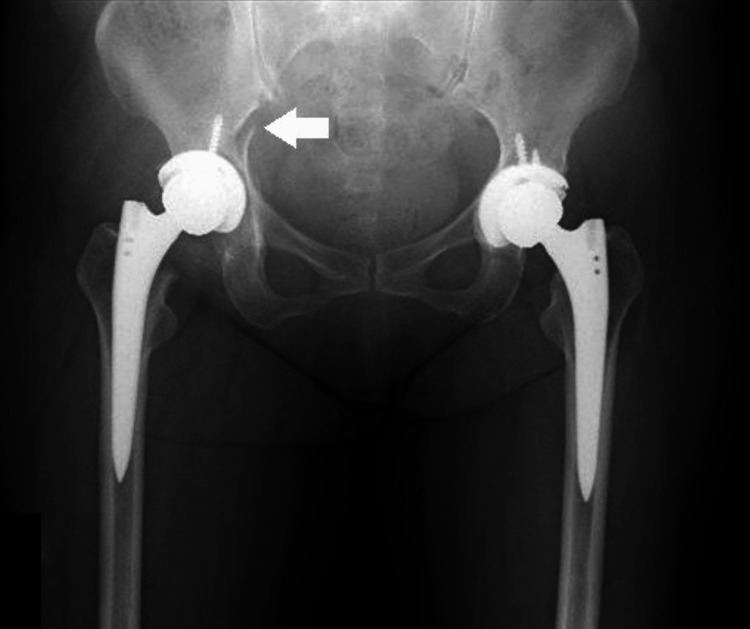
Plain radiograph of the pelvis at initial presentation (anteroposterior view). A right acetabular fracture was observed (arrow). There was no clear evidence of implant loosening.
